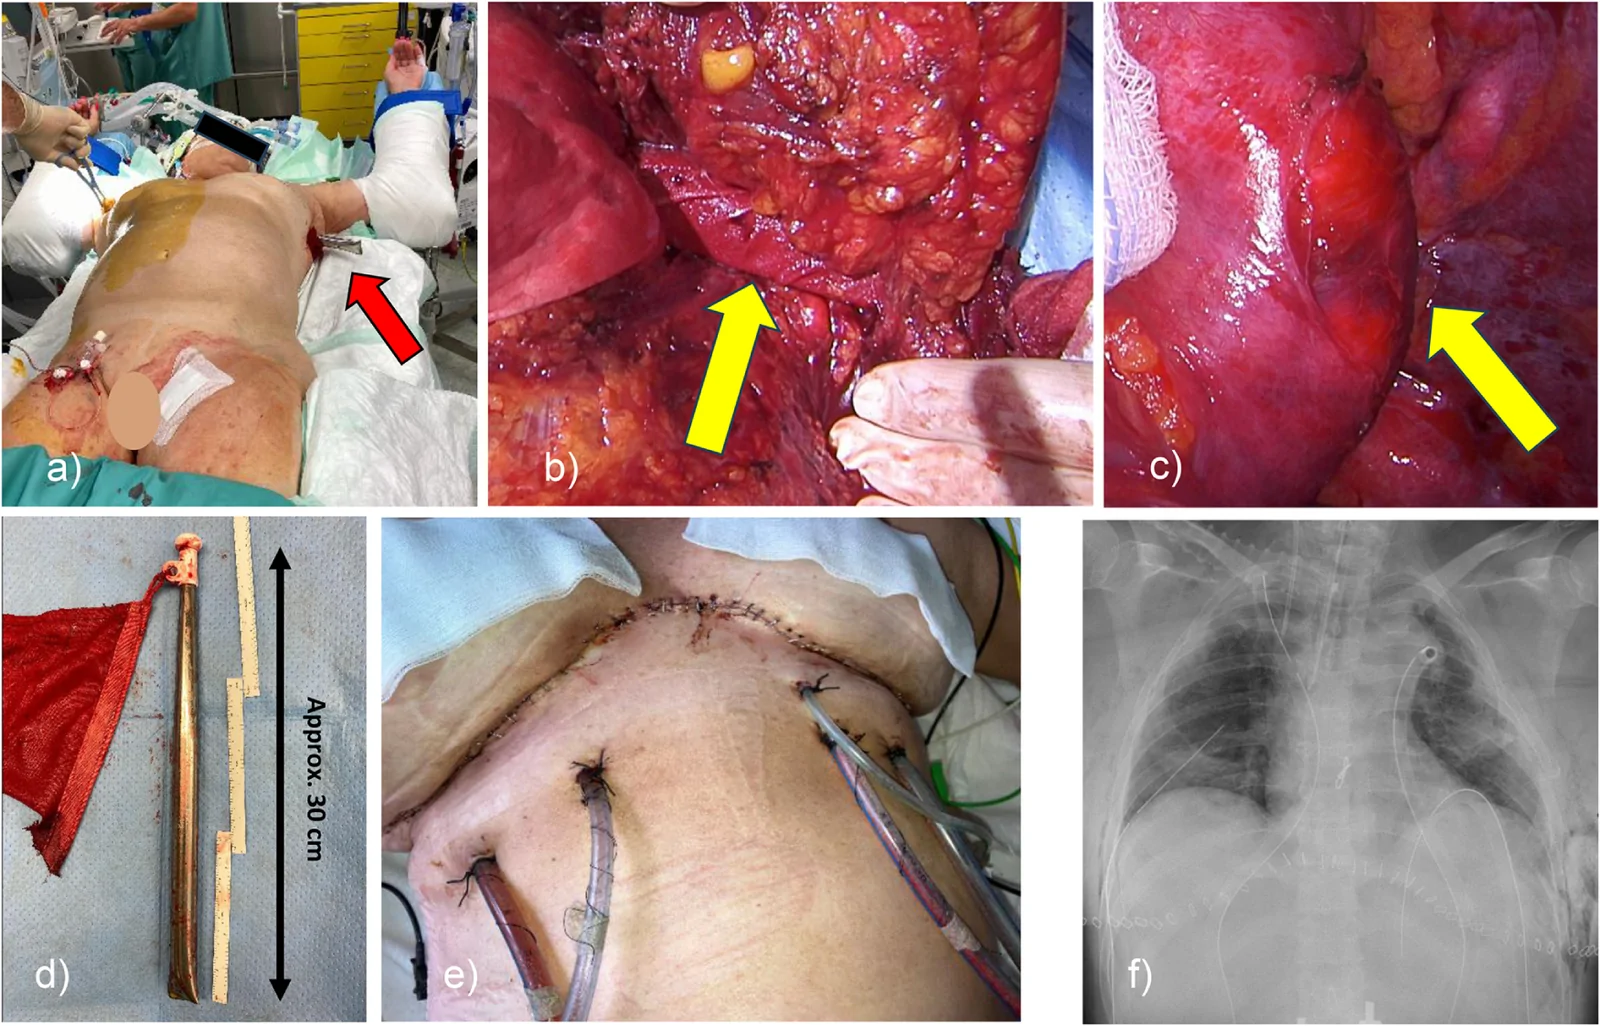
(a) The patient was positioned supine with both arms abducted; The red arrow indicates the shortened flagpole; Double-lumen endotracheal intubation was performed; right-sided femoral arterial and venous access was established for rapid cardiopulmonary bypass if required; and skin preparation was performed using a povidone-iodine solution. (b) Intraoperative image showing the flagpole and surrounding flag tissue (yellow arrow) during chest wall penetration; the left lung is on the left side of the screen. (c) Aortic wall after removal of the flagpole, showing a small adventitial laceration (yellow arrow). (d) Flagpole and flag tissue after removal. (e) Postoperative view of the surgical access, chest tubes, and vacuum drain. (f) Postoperative chest x-ray.
